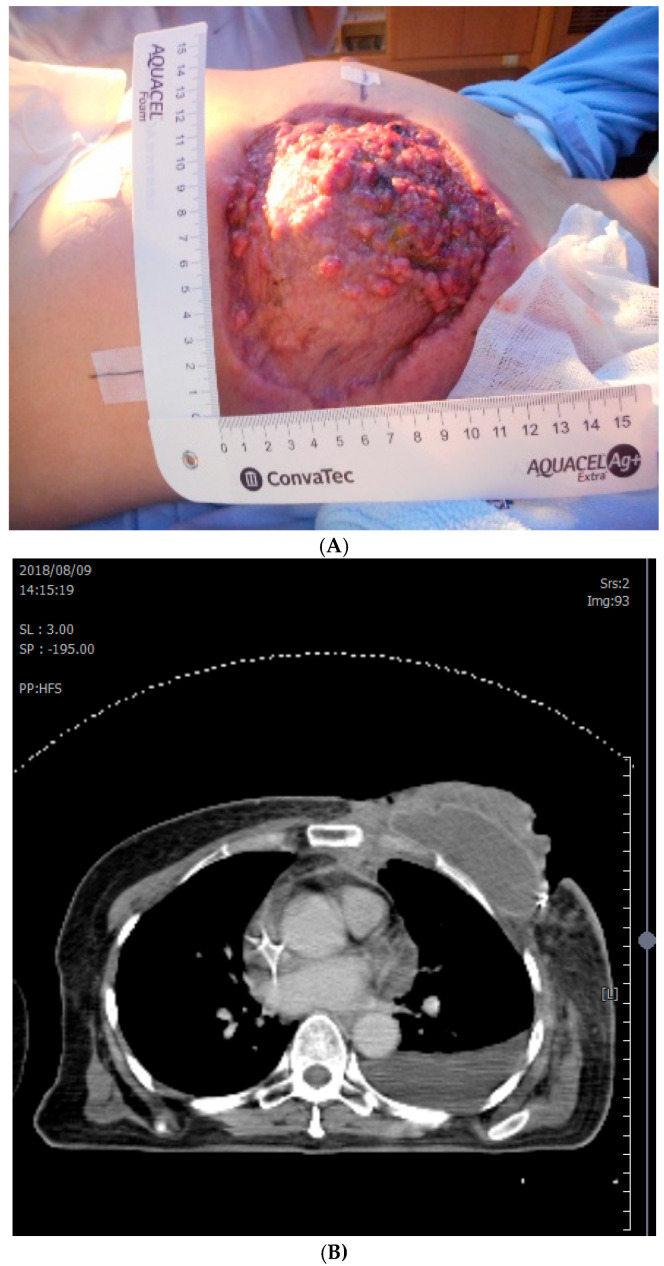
Clinical and radiological presentation of the aggressive chest wall recurrence. (A) Clinical image of the left chest wall tumor invading the whole skin above the left breast prosthesis, in August of 2018, just 2 months after second salvage surgery in June 2018. (B) CT image of the thick large tumor invading the whole skin above the left breast prosthesis and the nearby soft tissue close to the sternum, making the tumor unresectable and the prosthesis unremovable.
